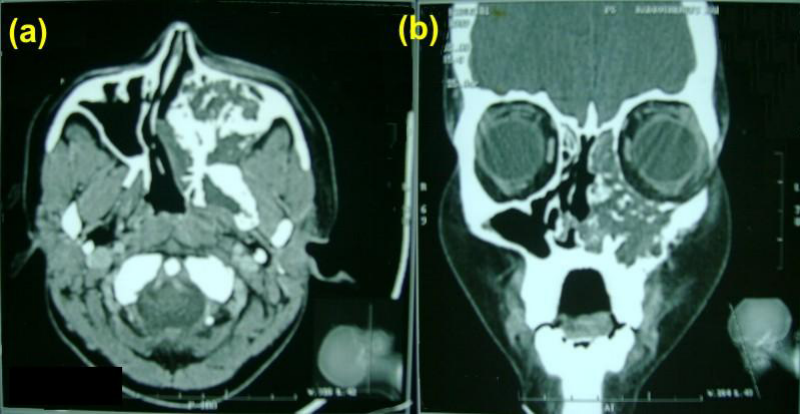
Axial and coronal contrast-enhanced paranasal sinuses and orbit CT-scan after 6 cycles of chemotherapy and radiotherapy (a, b). showing marked reduction in the tumor, the lesion is limited to the left maxillary sinus. A partial tumor remission was considered.
